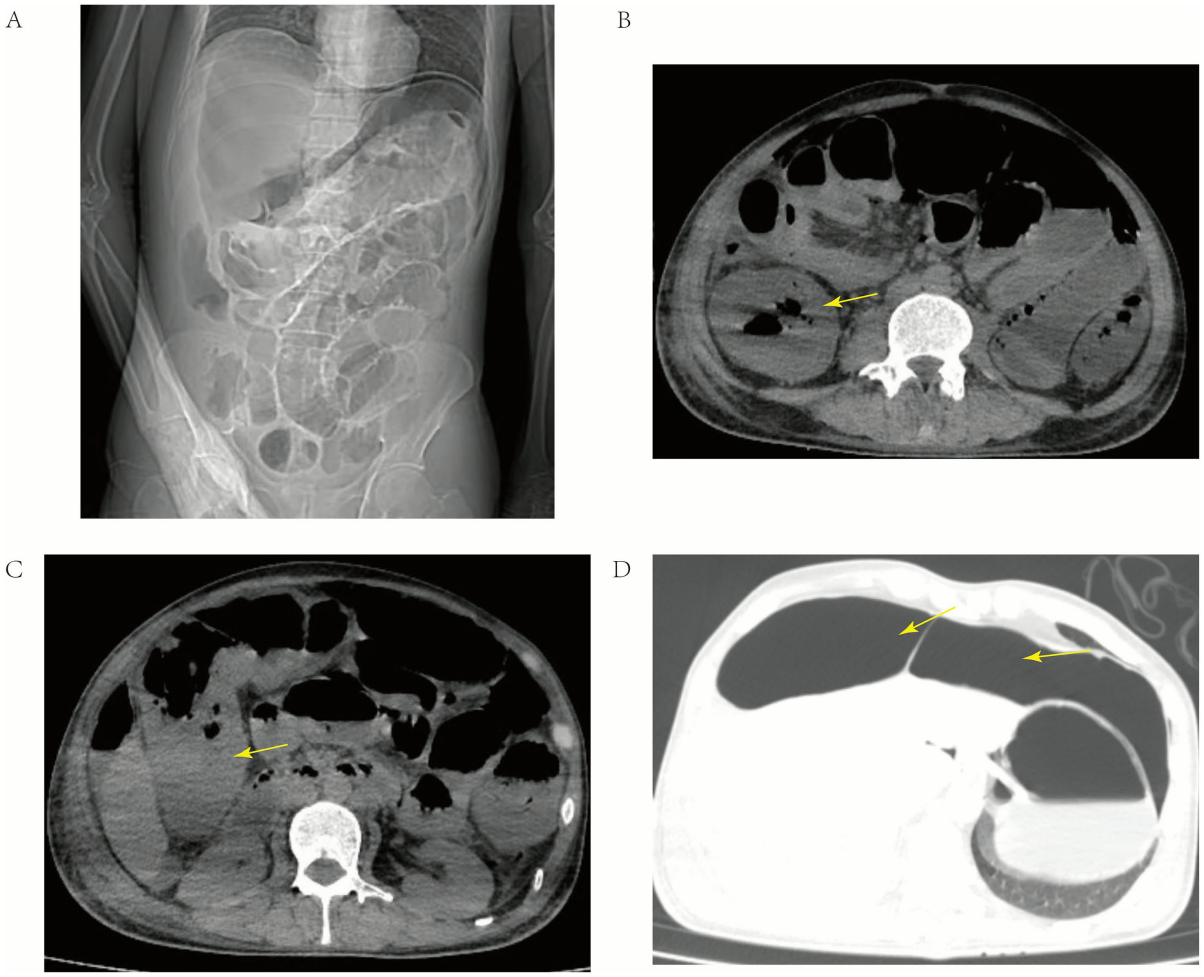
Preoperative abdominal CT images. (A) Visible abdominal bowel dilation with a small amount of free gas beneath the diaphragm. (B) Marked bowel wall edema, with the thickened ascending colon indicated by the yellow arrow. (C) Marked bowel wall edema, with the thickened hepatic flexure of the colon indicated by the yellow arrow. (D) Imaging under the lung window, showing free gas in the abdominal cavity, indicated by the yellow arrow.
